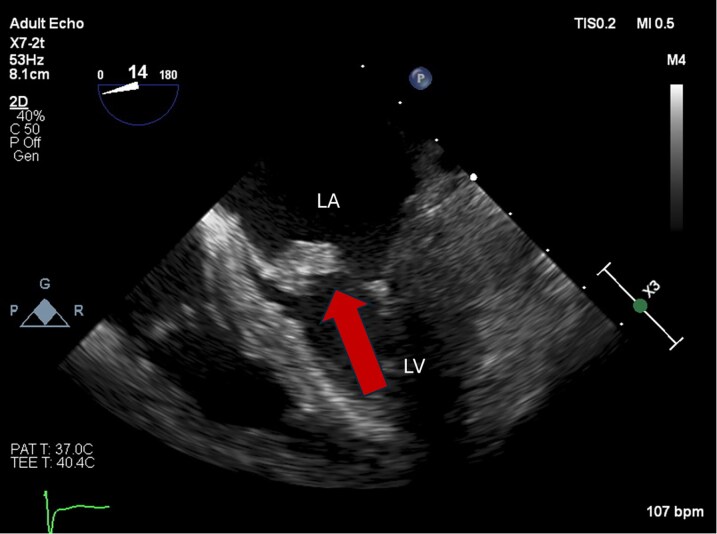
Transesophageal echocardiogram showing mitral valve vegetation.
